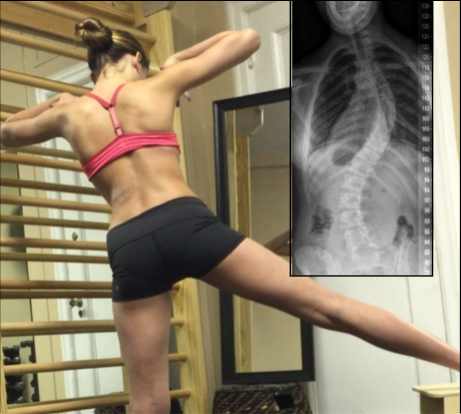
The “Muscle-Cylinder” exercise for major lumbar curves in patients with moderate-severe scoliosis (as seen in the radiograph) helps patients achieve spinal alignment and a corrected posture
